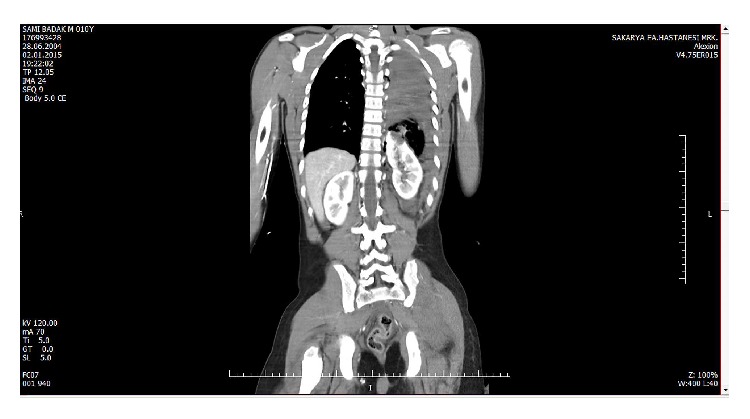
CT scan imaging of left diaphragmatic rupture and left intrathoracic kidney.
